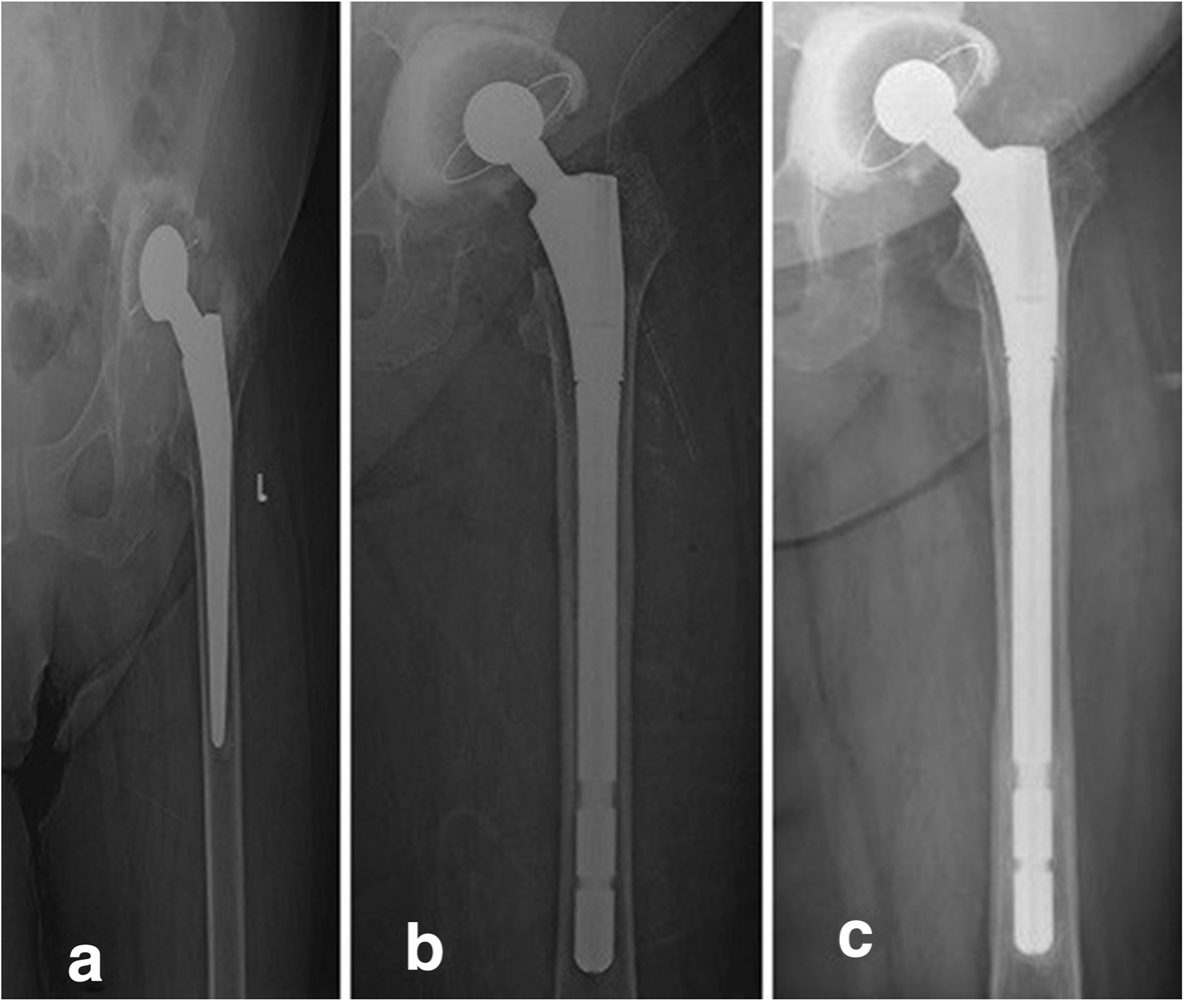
a Aseptic loosening of a cemented hip prosthesis implanted 18 years previously in a 68-year-old female. b Anteroposterior (AP) radiograph of the hip during the early postoperative period after revision with a modular type proximal porous-coated diaphyseal auto-locking femoral stem. c AP radiograph of the hip 72 months after revision
